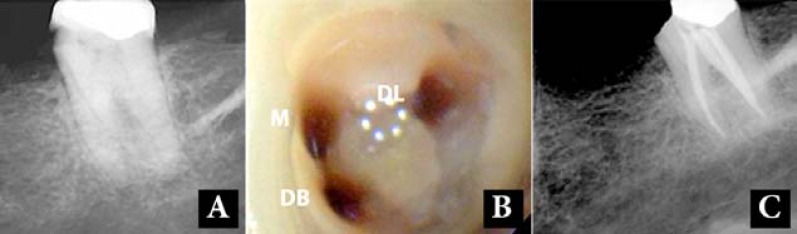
Case-3; *A)* Pre-operative radiograph shows the mandibular second molar. *B)* Two canals in mesial (M and DB) and one in distal (DL) of the chamber floor were detected. *C)* Post-operative radiograph shows that one mesial and two distal canals have been filled
